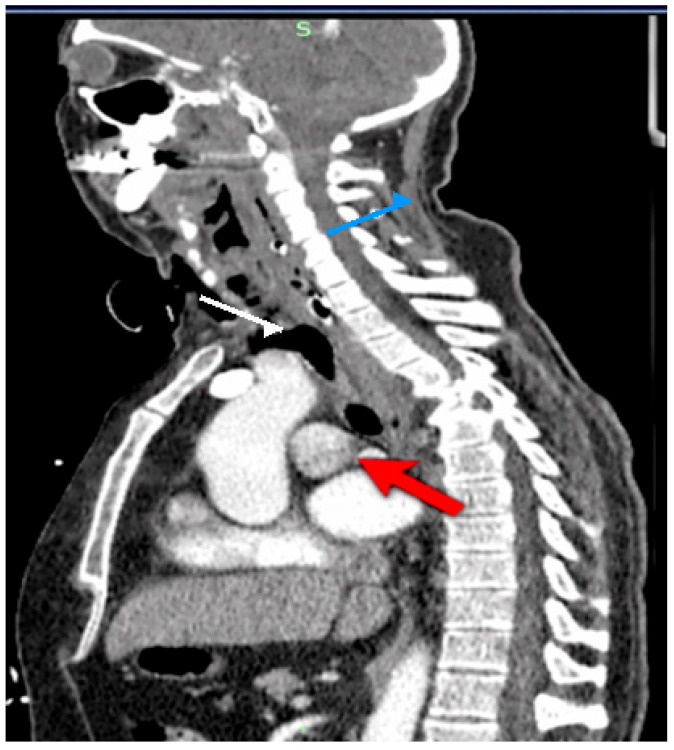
Contrast-enhanced CT scan (sagittal view) demonstrating a right paraesophageal fluid collection with associated gas bubbles and mediastinal extension. The red arrow indicates the paraesophageal fluid collection, the white arrow indicates intralesional gas bubbles, and the blue arrow indicates extension into the mediastinum, consistent with cervicomediastinal abscess formation secondary to esophageal perforation.
